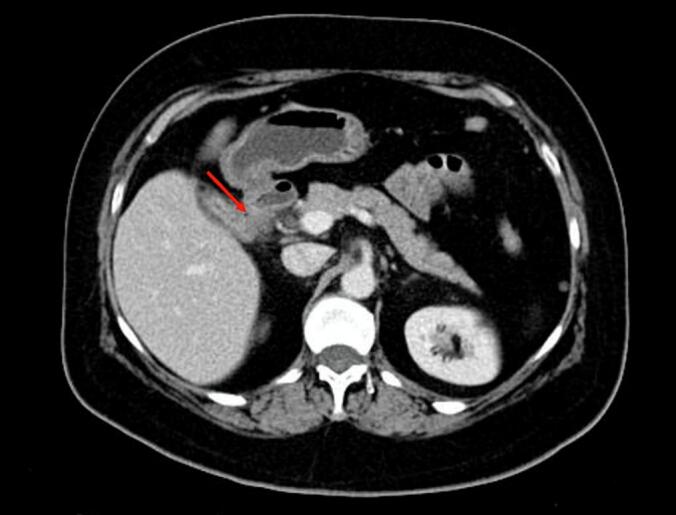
Axial abdominal CT scan view suggesting the cholecysto-duodenal fistula (red arrow). (For interpretation of the references to colour in this figure legend, the reader is referred to the web version of this article.)
